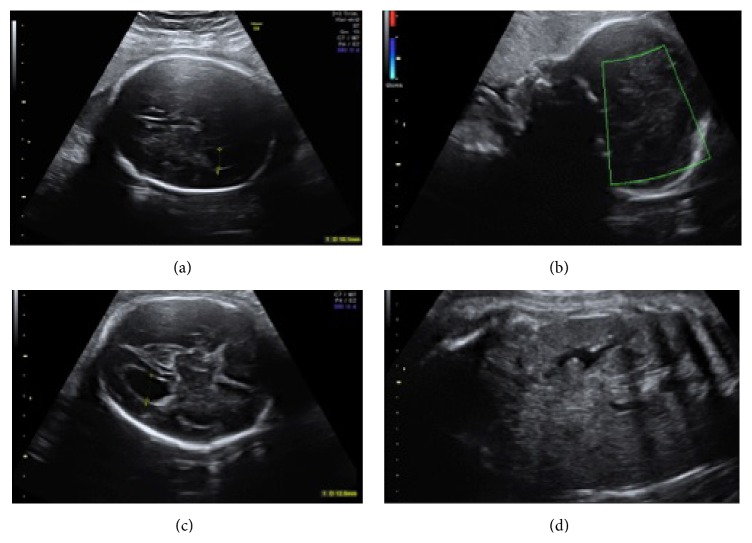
Ultrasound images of absent corpus callosum, mild ventriculomegaly, and enlarged kidney. (a) US image of absent cavum septum pellucidum. (b) US image of absent corpus callosum. (c) US image of ventriculomegaly. (d) US image of enlarged kidney.
